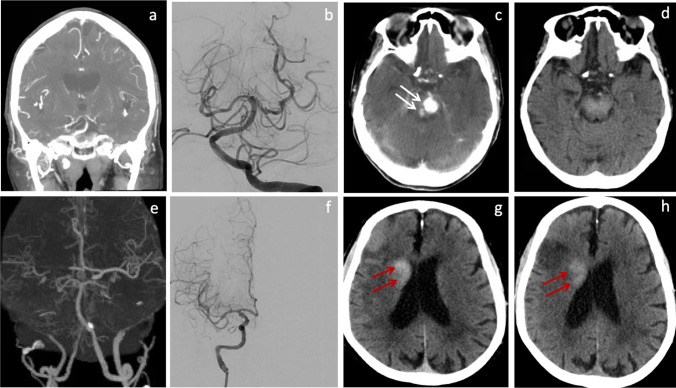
Upper panel: contrast extravasation (white arrow). A 75-year-old patient presented with drowsiness and speech difficulty. CTA showed distal BA occlusion (a). Immediately after endovascular recanalization (b), CT scan showed hyperdense lesions in the pons (c), which resolved on follow-up CT scan 24 h later (d). Lower panel: ICH (red arrows). A 74-year-old patient presented with slurred speech and left-sided weakness. CTA sowed right carotid terminus and MCA occlusion (e). Immediately after endovascular recanalization (f), CT scan showed hyperdense lesion in the right basal ganglia (g), which persisted on follow-up CT scan 24 h later (h)
